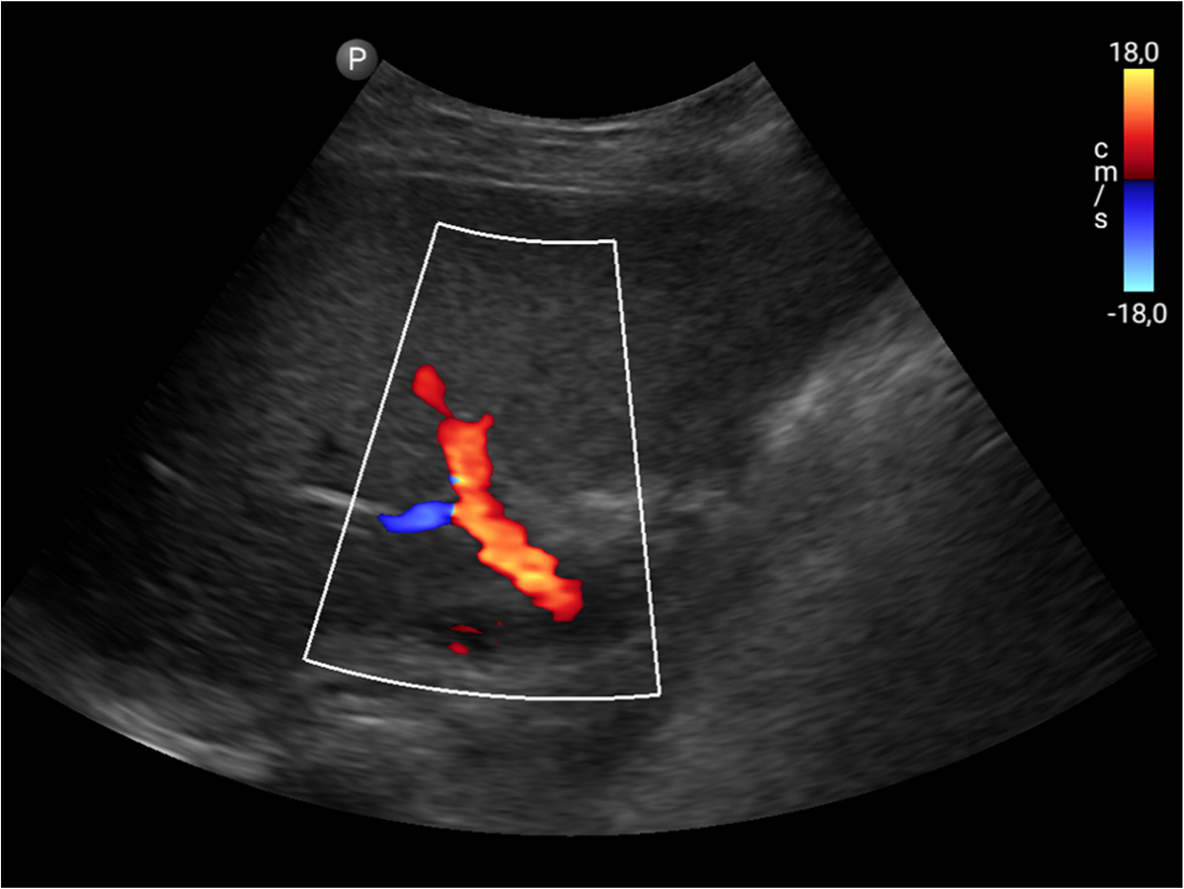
Philips Lumify curved array transducer: Colour Doppler imaging of the portal vein
